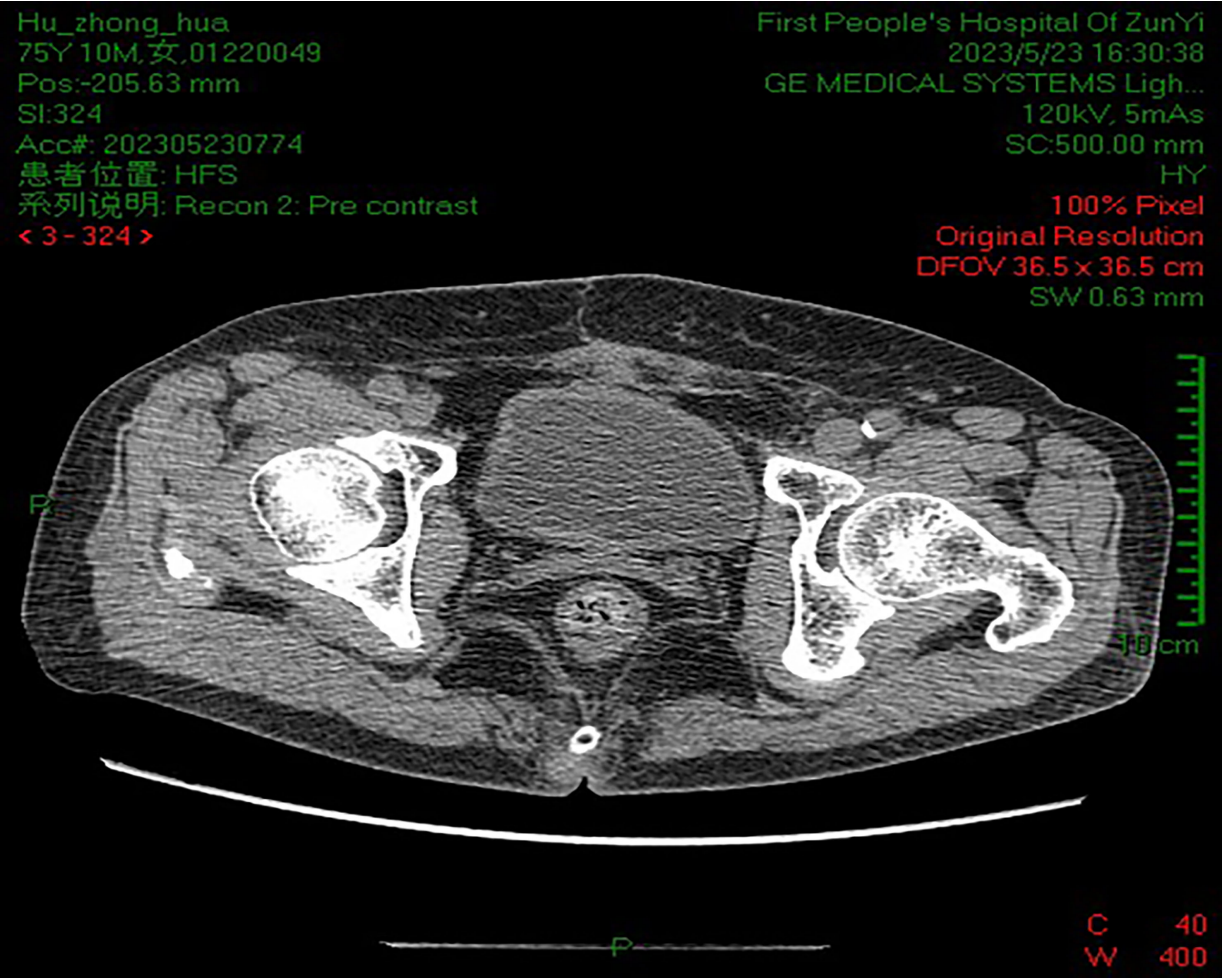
Pelvic CT did not show tumor recurrence and metastasis.
